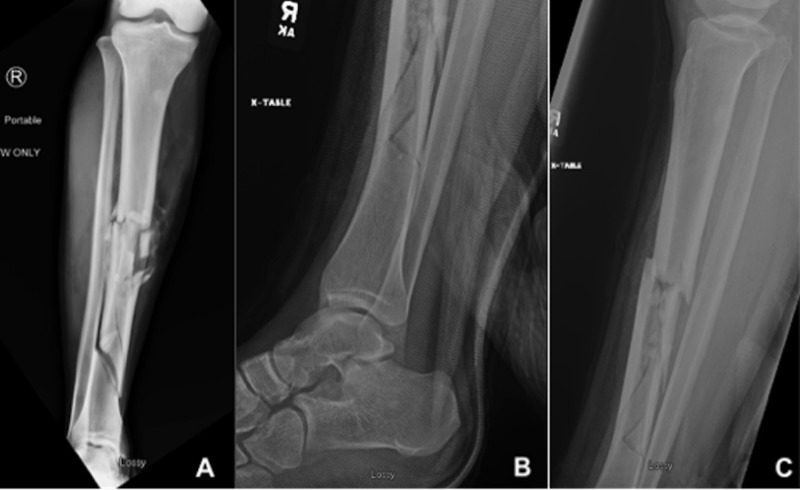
Injury radiographs (A) Anteroposterior radiograph of the right tibia and fibula, (B) lateral radiograph of the right ankle, (C) lateral radiograph of the right tibia and fibula.
